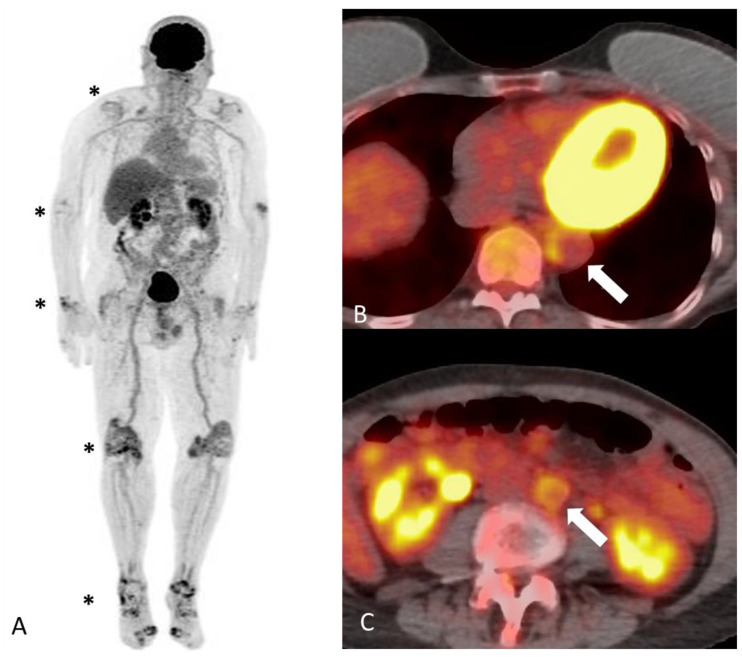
A 78-year-old man with polymyalgia rheumatica and active vasculitis. Maximum intensity projection images of FDG PET (A) demonstrating abnormal radiotracer accumulation in multiple joints (shoulders, elbows, hands, hips, knees and feet) in keeping with polyarticular inflammatory arthropathy (asterisks). Axial images of fused PET CT at the level of the descending aorta (B) and abdominal aorta (C) showing near concentric tracer avid mural thickening to the aorta (arrows), most consistent with active vasculitis.
